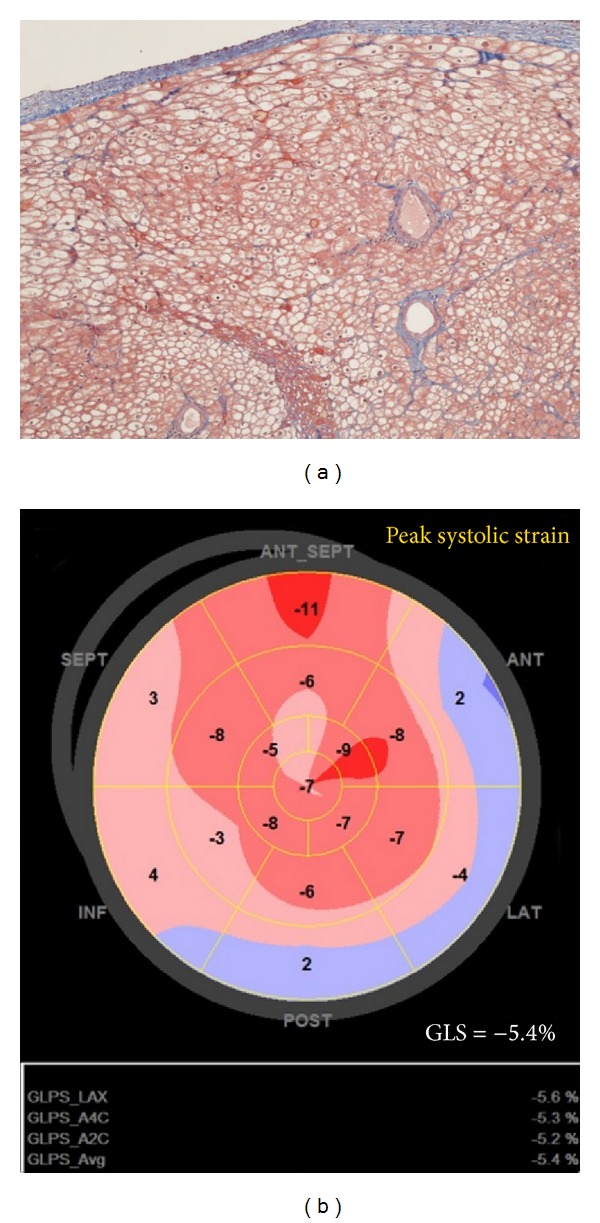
(a) Endomyocardial biopsy, cardiac explant 11.01.2011, right ventricle, and Masson's trichrome ×125, showing myocytolysis and thrombotic occlusion of minor arterial branches. (b) Echocardiography 06.01.2011: bulls plot of global longitudinal strain = −5.4%. LV-EF at this point 49% (LV-EDV 82 mL, LV-ESV 42 mL).
